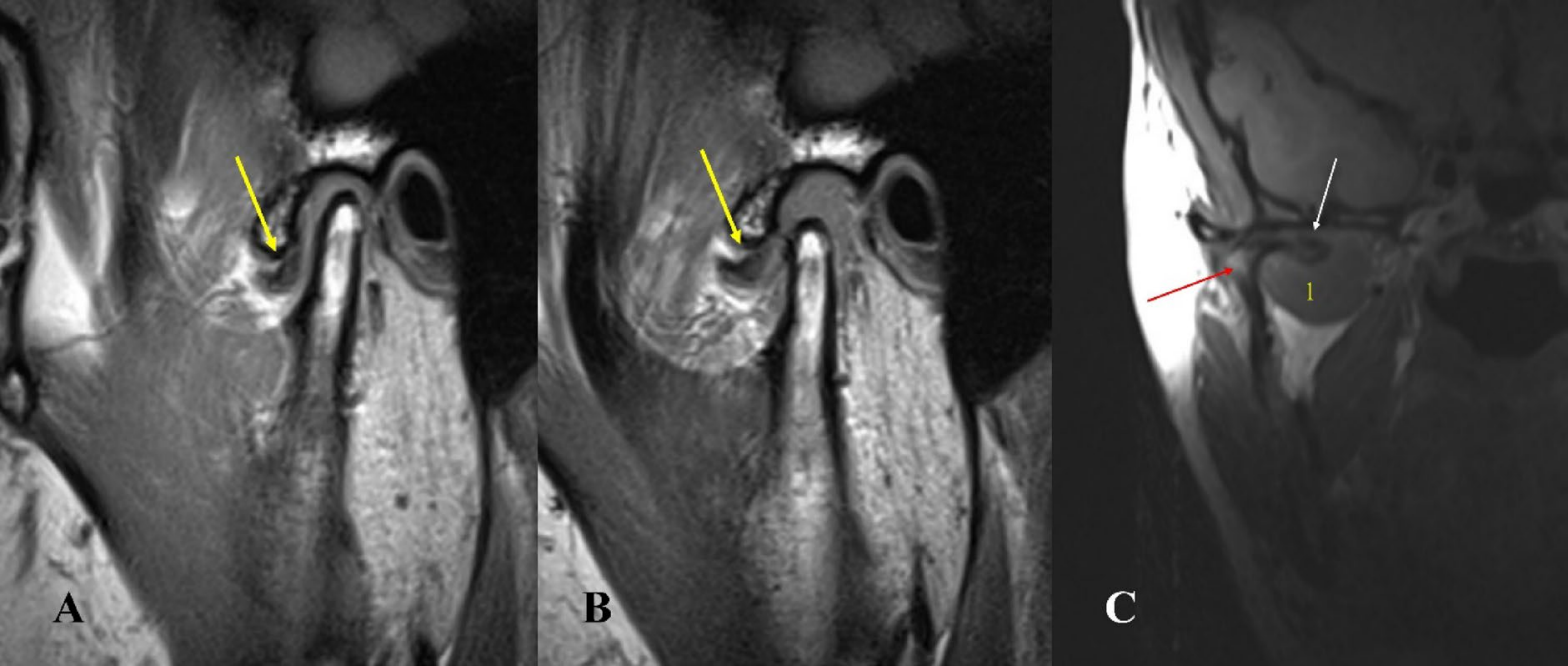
Oblique sagittal T1-weighted images represent Type I with one head of lateral pterygoid muscle head attached to the DCC associated with disc displacement without reduction and severe form of osteoarthritis.
